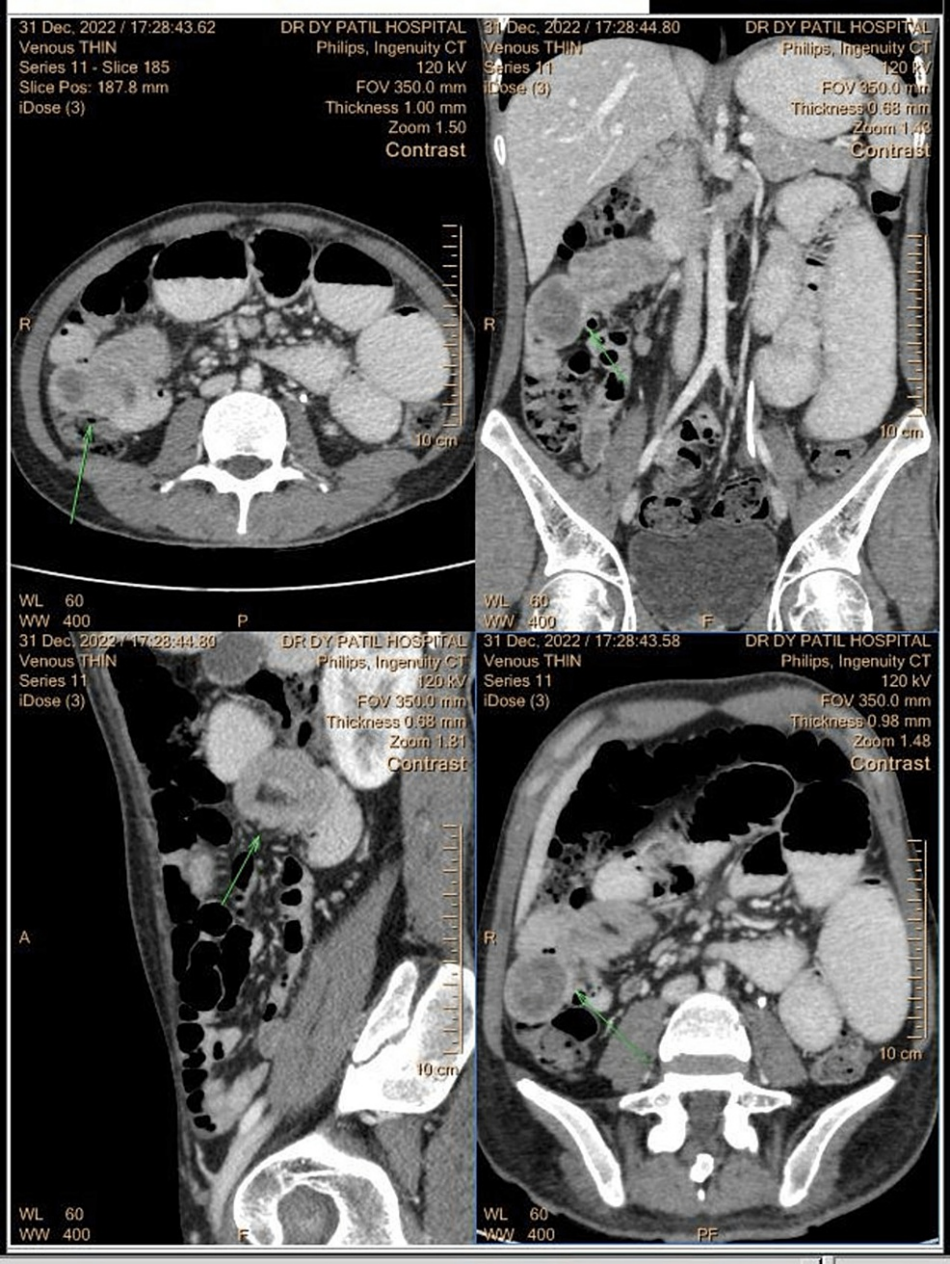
CECT Abdomen showing moderate to severe dilatation of jejunum due to hypodense enhancing amorphous lesion arising from jejunum with jejuno-jejunal intussusception (marked with green arrow). CECT: contrast-enhanced computed tomography
